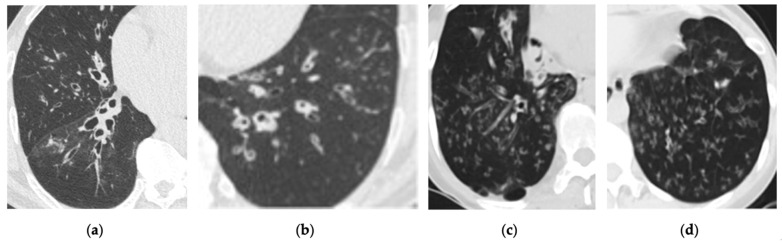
Incidental finding of bronchiectasis with centrilobular nodules associated with tree-in-bud pattern on low-dose computed tomography (LDCT) scan. (a,b) Axial LDCT scans of a 65-year-old male with chronic cough demonstrate bronchiectasis and findings of infectious bronchiolitis. (c,d) Axial LDCT scans of a 42-year-old female reveal similar findings in the bilateral lower lungs.
